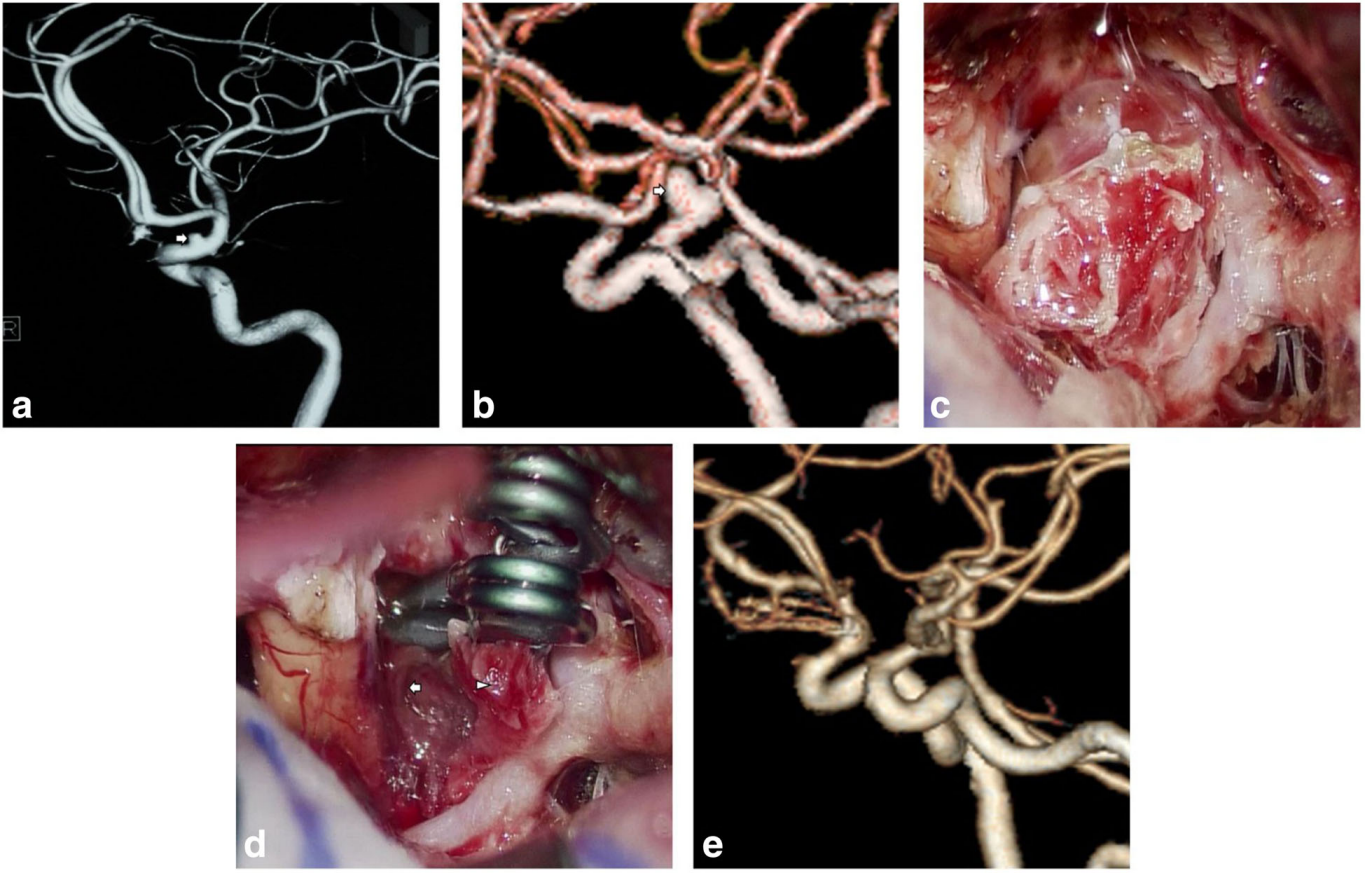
Case 5. a, b Preoperative angiography demonstrated a tapered BLA and its rapid growth into saccular configuration (white arrow). c This anteriorly projecting aneurysm was tightly adhered to the frontal lobe. d The aneurysm was composed of a thickened false membrane (arrowhead) and a much thinner true wall (arrow). e Postoperative CTA showed complete obliteration of the aneurysm
